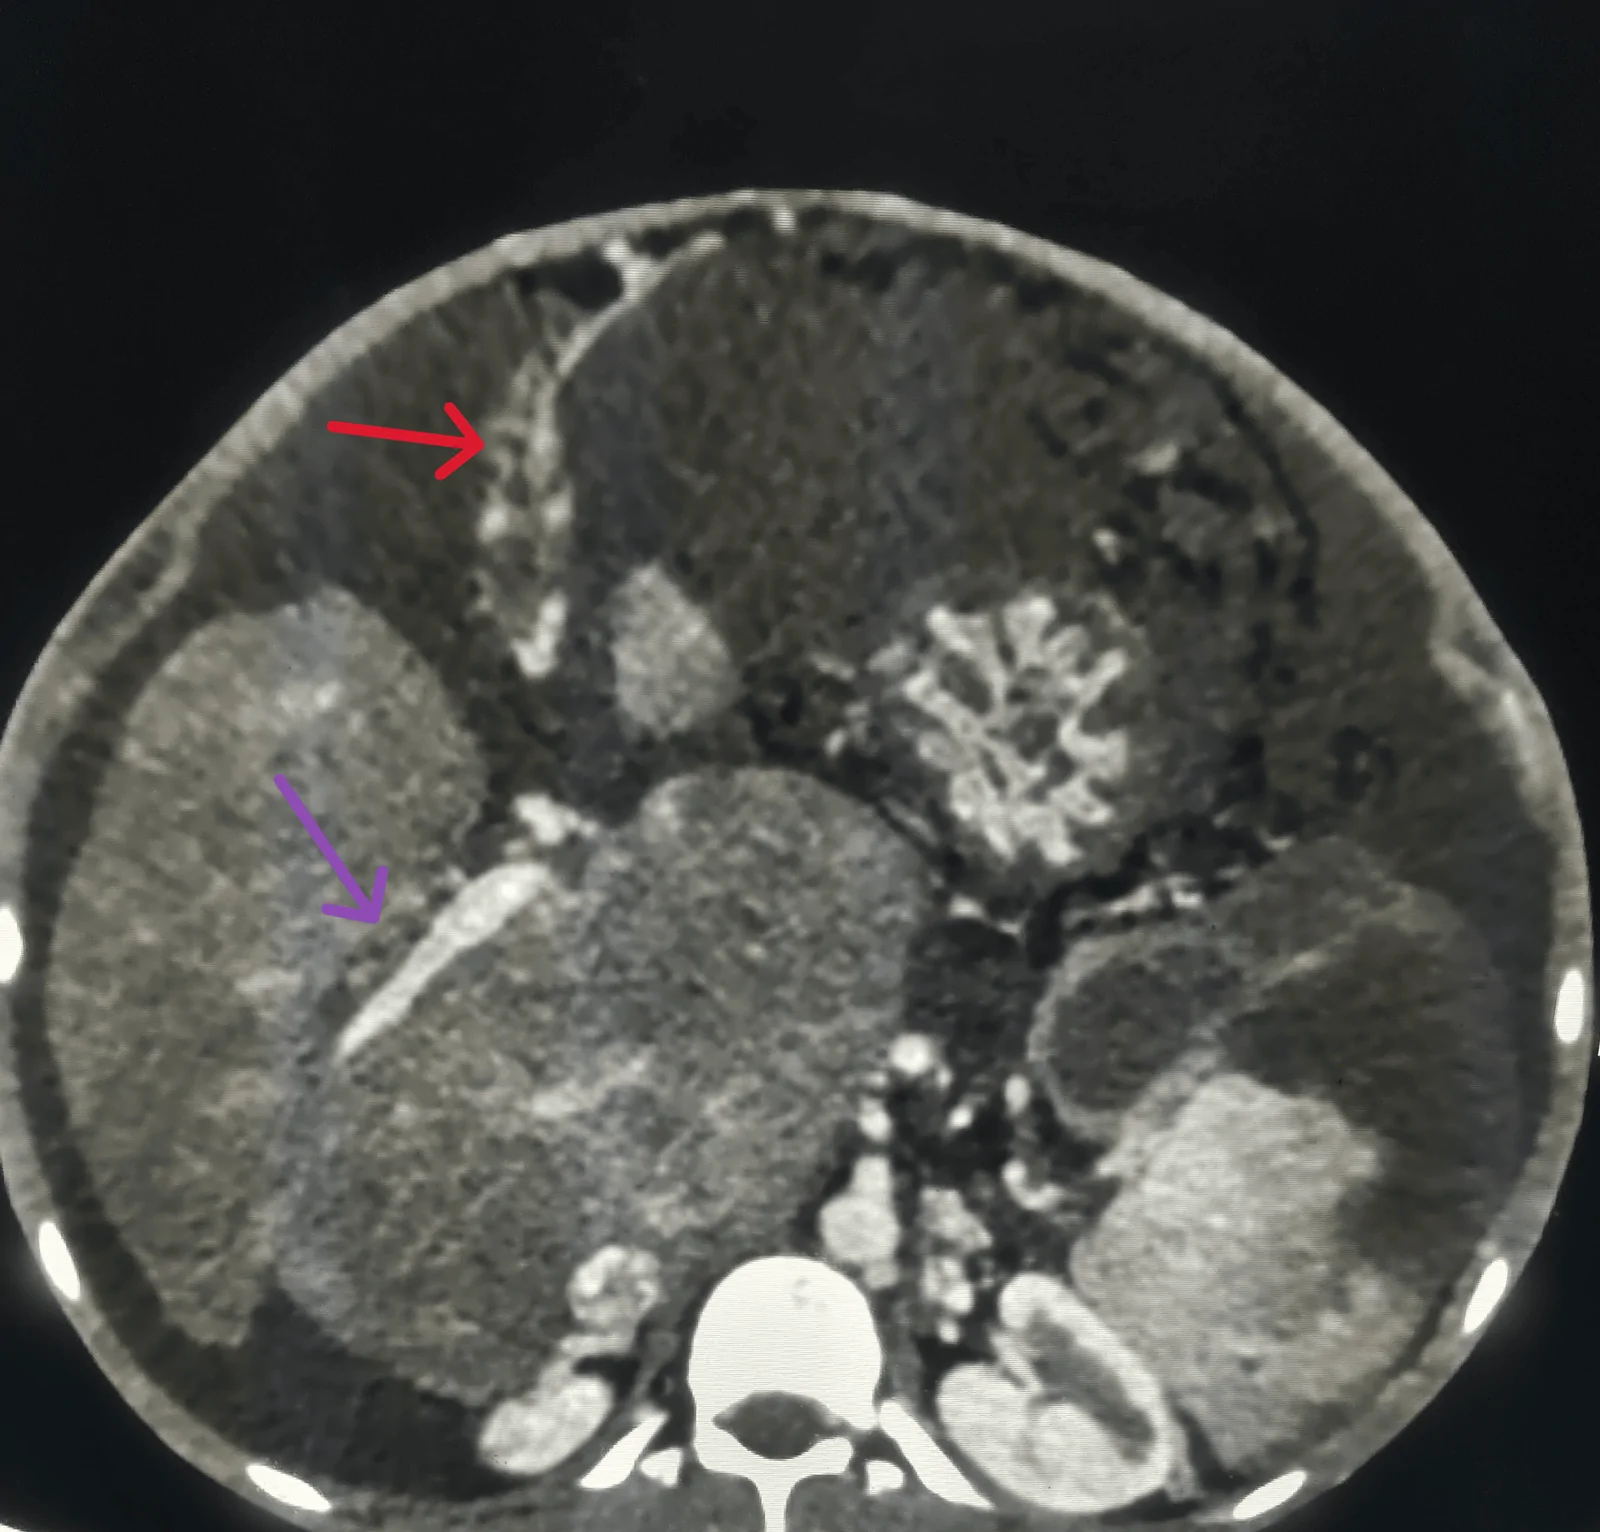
Axial contrast-enhanced CT section of the upper abdomen (hepatic region) showing recanalisation of the paraumbilical vein (red arrow) and visualisation of only a single portal vein branch (purple arrow), consistent with portal hypertension.
